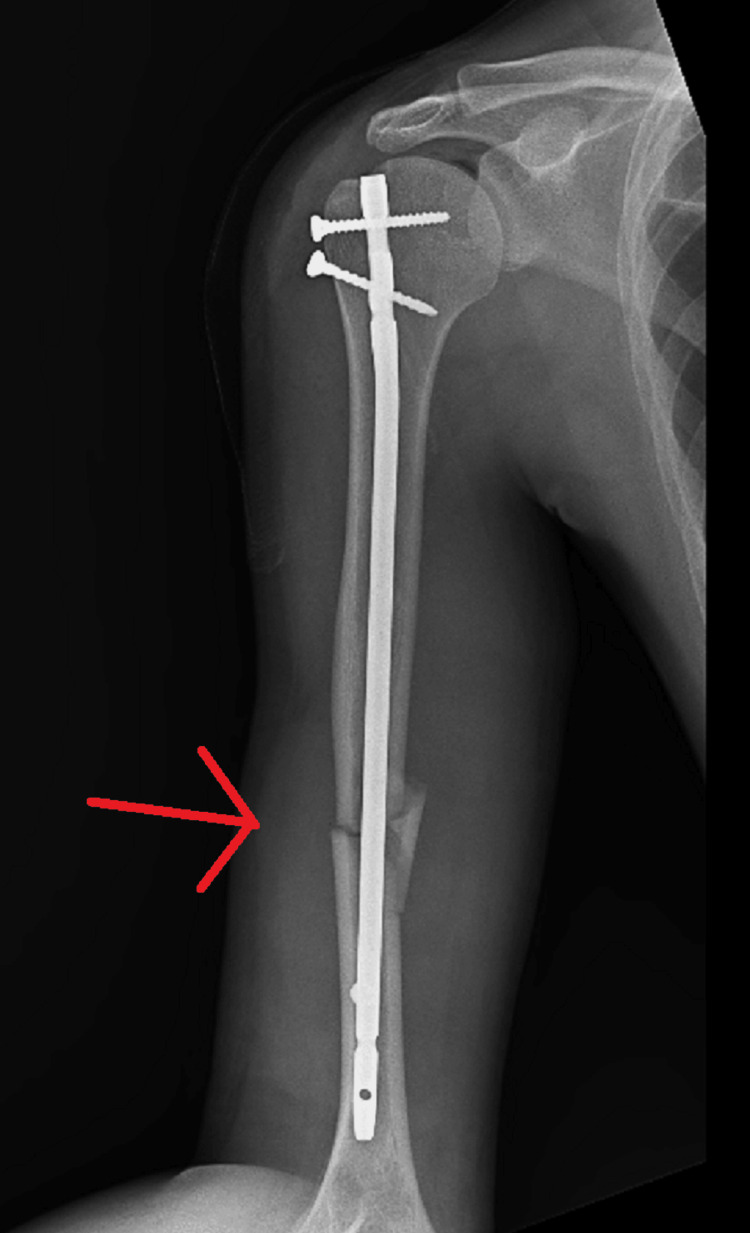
Anterior-posterior radiograph showing CRIF with intramedullary nailing for mid-shaft humerus fracture on the right side CRIF - closed reduction and internal fixation
